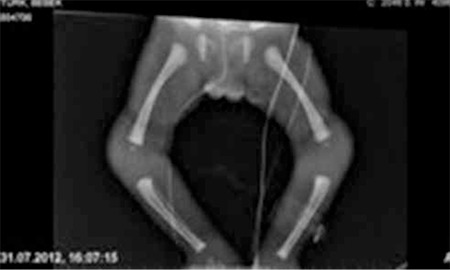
No skeletal abnormalities on X-ray image
